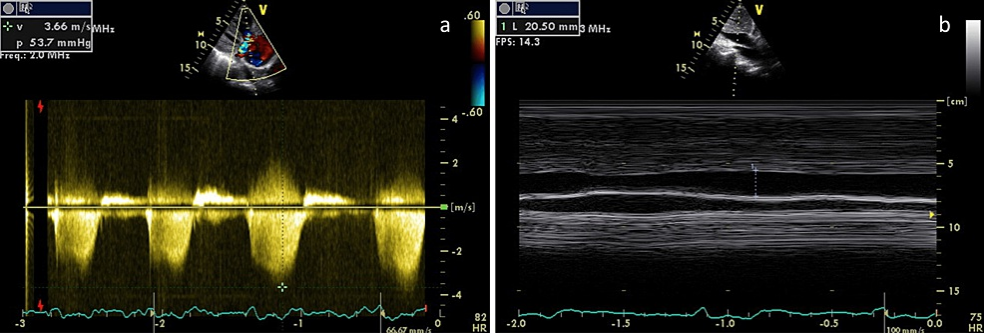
Estimated pulmonary arterial systolic pressure before Graves' treatment Echocardiographic parameters, before Graves’ treatment, showing estimated pulmonary arterial systolic pressure of 69 mmHg. Systolic artery pressure was estimated from a) tricuspid regurgitant jet velocity of 3.66 m/s and calculated by Bernoulli equation maximum gradient of 54 mmHg plus b) estimated right atrial pressure of 15 mmHg (slightly dilated inferior vena cava with respiratory variation < 50%).
